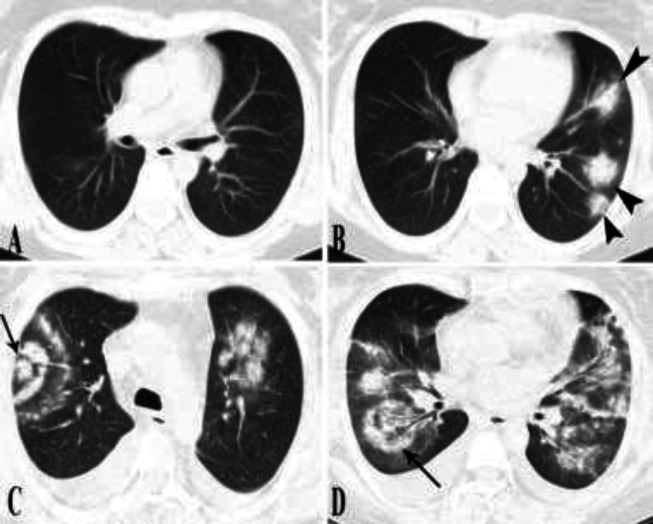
Serial imaging of case 7. Initial CT scan (A, B) depicts rounded consolidative opacities (arrowheads). One week later, two foci of target appearance developed in the right upper lobe and superior segment of right lower lobe (arrows). Mild cardiomegaly and bilateral pleural effusion are also present
